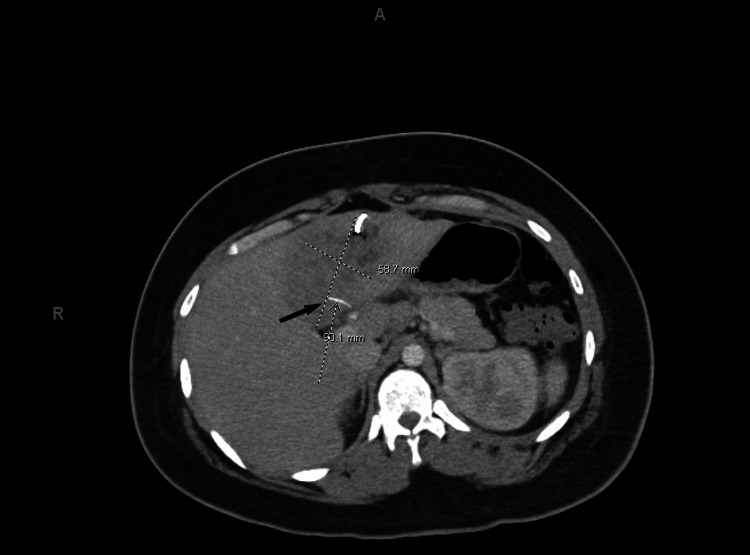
Repeat computed tomography (CT) of the abdomen. Repeat spiral CT abdomen obtained six days after initial imaging. Imaging was obtained after the placement of a pigtail catheter seen in the most anterior aspect of the hepatic collection. The original abscess was still present and only minimally smaller despite drainage. Now, the formation of a small, new abscess was noted in the anterior peritoneum that abuts the rectus abdominis muscle. Lastly, unchanged curvilinear hyperdensity (black arrow) was still present within the collection, concerning for a migrated foreign body as the source of the abscess.
